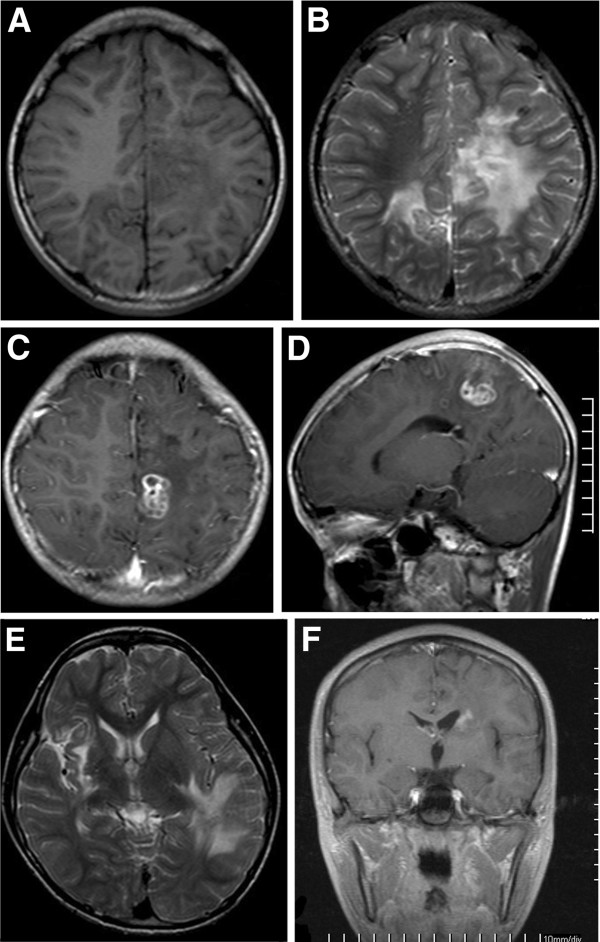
MR images for the case 8. A. The T1W image shows there are two white matter irregular patchy with hypointensity in the parietal lobes. B. The T2W images shows there are two white matter irregular patchy with hyperintensity in the parietal lobes. C and D. The enhanced T1W images show a beaded enhancement for the lesion. E and F. The images from the MR follow-up scans after 5 months shows the location and shape for the enhancing lesion was changed (C and E).
